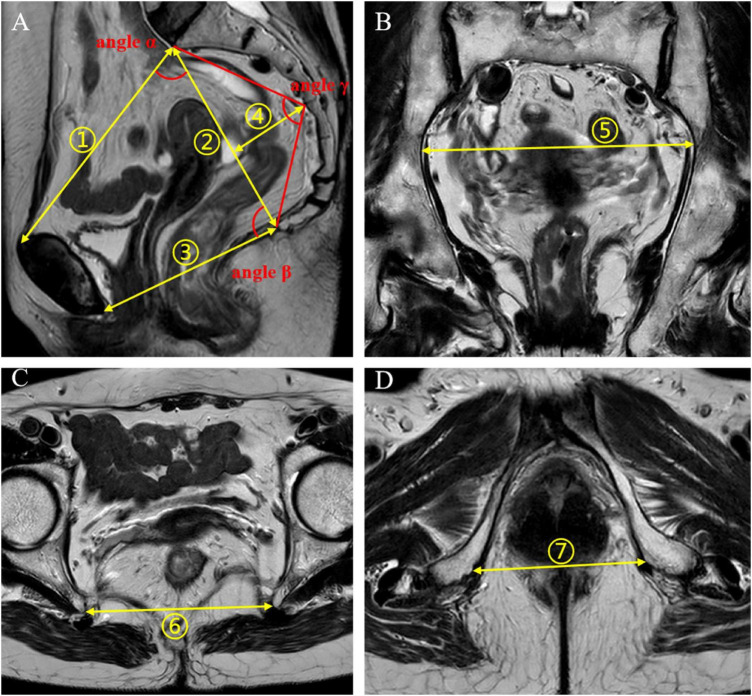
Images showing the MRI-based pelvimetric parameters. (A) Sagittal MRI showing ➀the pelvic inlet (distance between sacral promontory and superior aspect of pubic symphysis), ➁the pelvic depth (distance between sacral promontory and tip of the coccyx), ➂the pelvic outlet (distance between tip of the coccyx and inferior aspect of pubic symphysis), and ➃the sacral depth (perpendicular distance between pelvic depth and the deepest portion of the sacrococcygeal hollow) and the pelvic angles (α, β, γ) (α: angle between pelvic inlet and the pelvic depth; β: angle between the pelvic outlet and the pelvic depth; γ: angle between sacral promontory and the tip of the coccyx based on the deepest sacrococcygeal point). (B) Coronal MRI showing ➄the transverse diameter (the maximum distance between the bilateral iliopectineal lines). (C) Axial MRI showing ➅the interspinous distance (distance between tips of ischial spines). (D) Axial MRI showing ➆intertuberous distance (distance between the lowest points of the ischial tuberosities).
